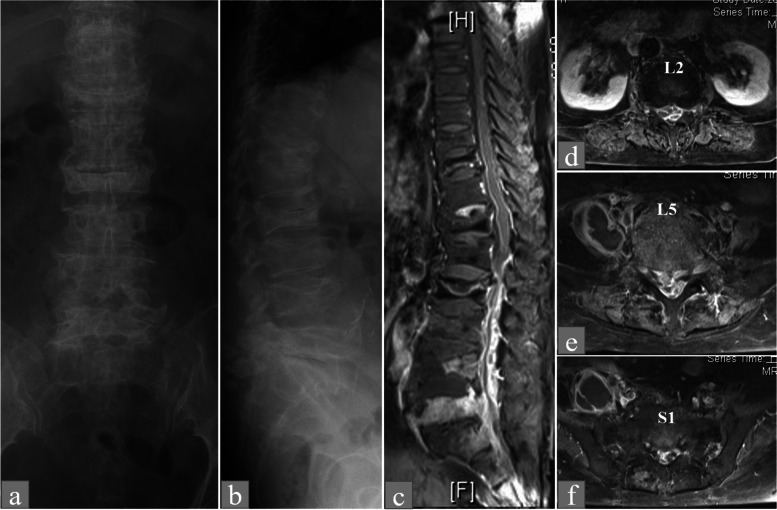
71-year-old female (No.7) with anaerobic spondylodiscitis presenting with multiple vertebral body compression fractures. A Lumbar anteroposterior view displaying multiple vertebral body compression fractures. B Intervertebral cleft within an L2 vertebral body compression fracture in the lumbar lateral and anteroposterior view. C Sagittal gadolinium-enhanced fat-suppressed T1-weighted imaging of abscess formation within the L2 vertebral body, and osteomyelitis in the L4 and L5 vertebral bodies with purulent collection in the intervertebral space (D, E, F) Contrast-enhanced T1-weighted axial imaging of a circumferential spinal epidural abscess observed at L2–S1 levels and a right-side iliopsoas muscle abscess
